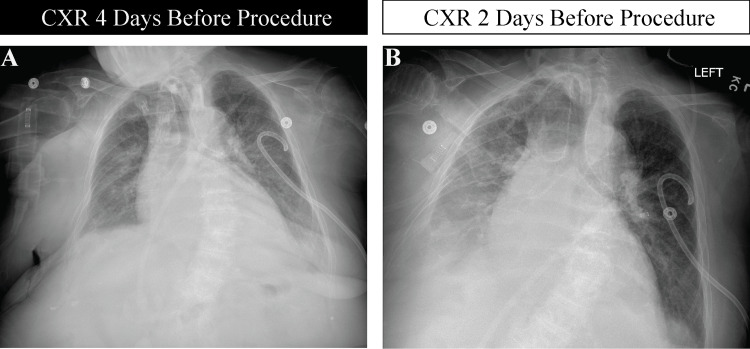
Chest radiographs obtained four and two days prior to the procedure Portable anteroposterior (AP) chest radiographs obtained four days (A) and two days (B) prior to the procedure. The radiographs demonstrate post-tracheostomy status with the tracheostomy tube tip projecting approximately 5.5 cm above the carina. Both images demonstrate moderate cardiomegaly with bilateral pulmonary vascular congestion, a moderate right pleural effusion, and a mild left pleural effusion with adjacent atelectasis. A left pleural pigtail catheter is visualized. Compared with the prior radiograph (A), radiograph B demonstrates interval progression of the right pleural effusion. CXR - chest X-ray
